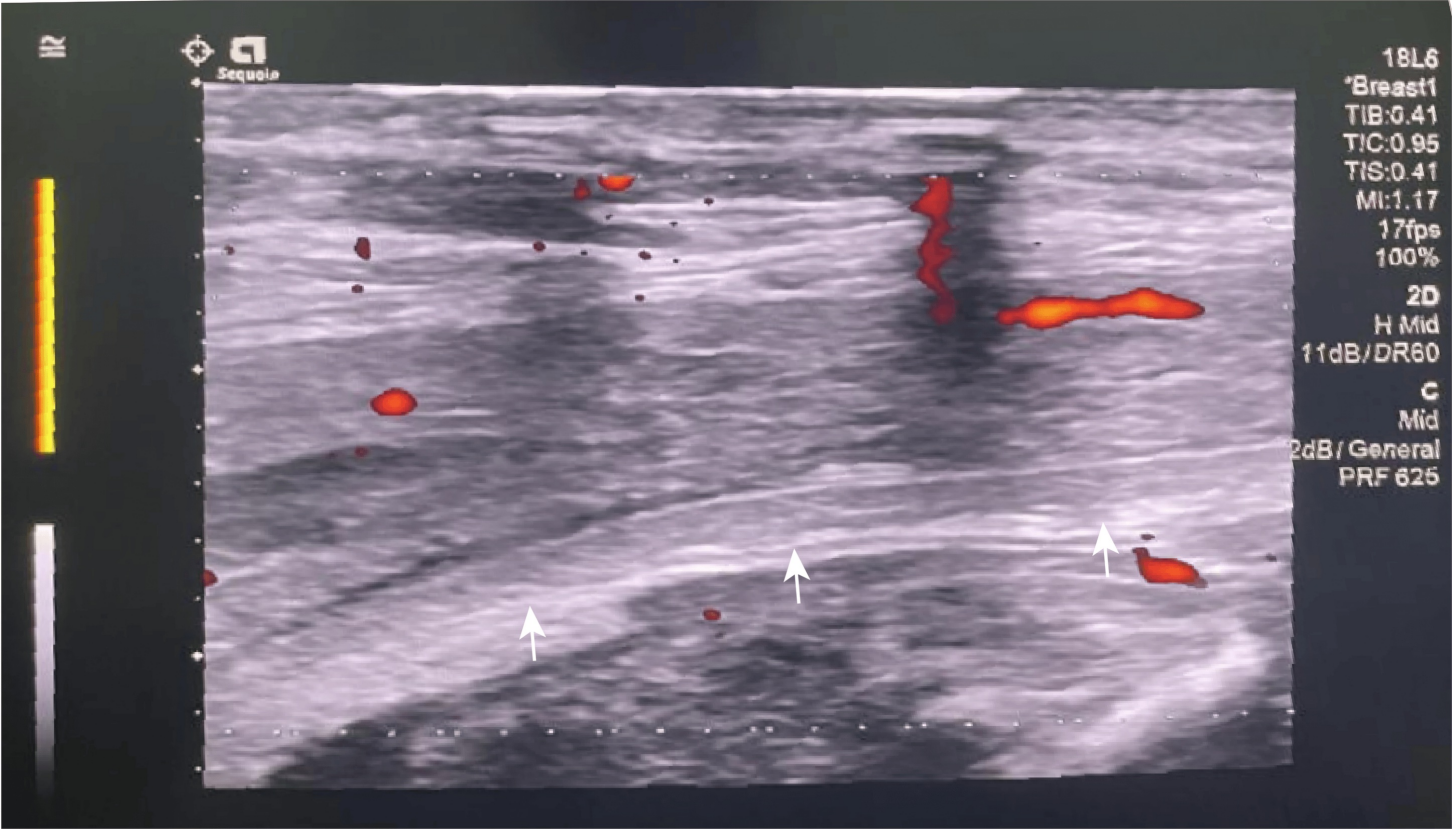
Ultrasound of the abdominal wall in the area of the cesarean section approach showed no venous malformations. The myometrium and peritoneum are not involved (indicated by the white arrows).
